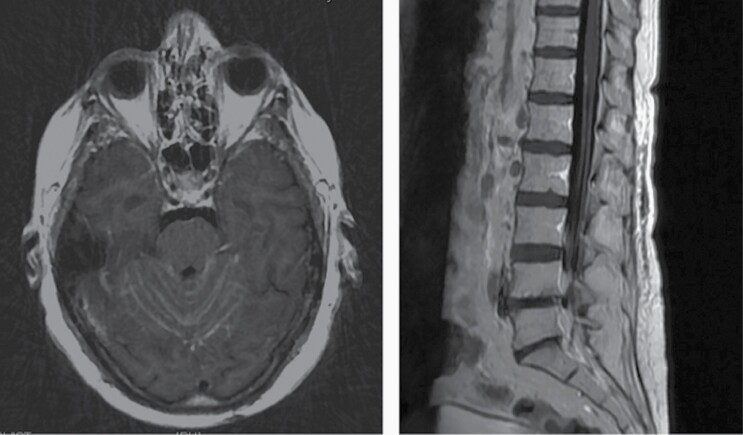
Radiographic appearance of leptomeningeal metastasis. Fifty-five year old RH woman with BRCA1 germline mutation and new onset ataxia.
